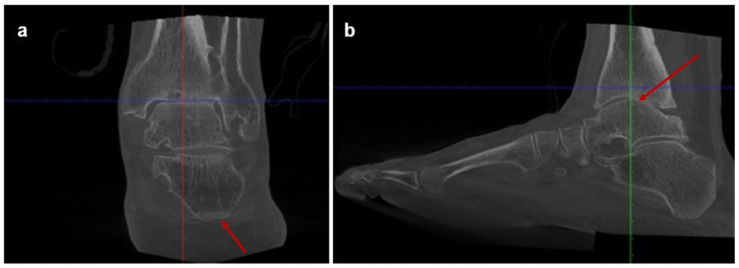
Preoperative weight-bearing computed tomography (WBCT) scans of Case two: (a) coronal and (b) sagittal views showing severe tibiotalar osteoarthritis. The arrow in panel (a) indicates valgus malalignment, while the arrow in panel (b) indicates posterior talar displacement.
